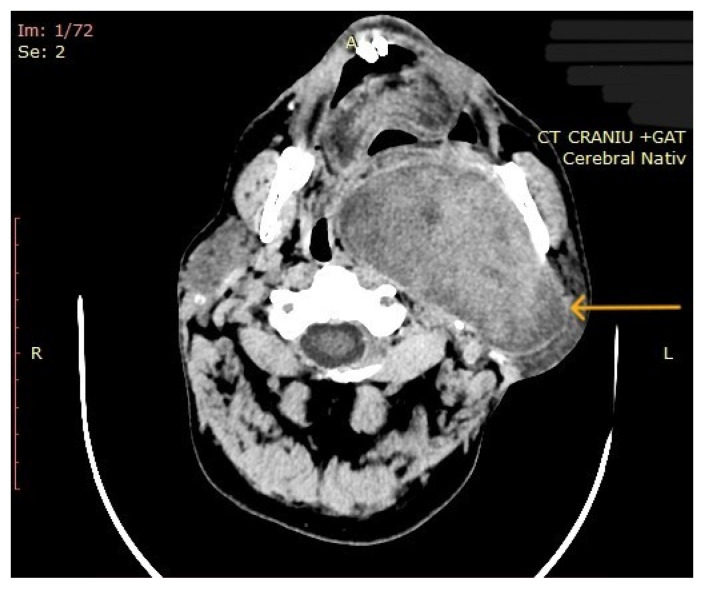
CT imaging: a mass with inhomogeneous uptake of contrast, developed from the left parotid lodge (the arrow represents the tumor mass).
